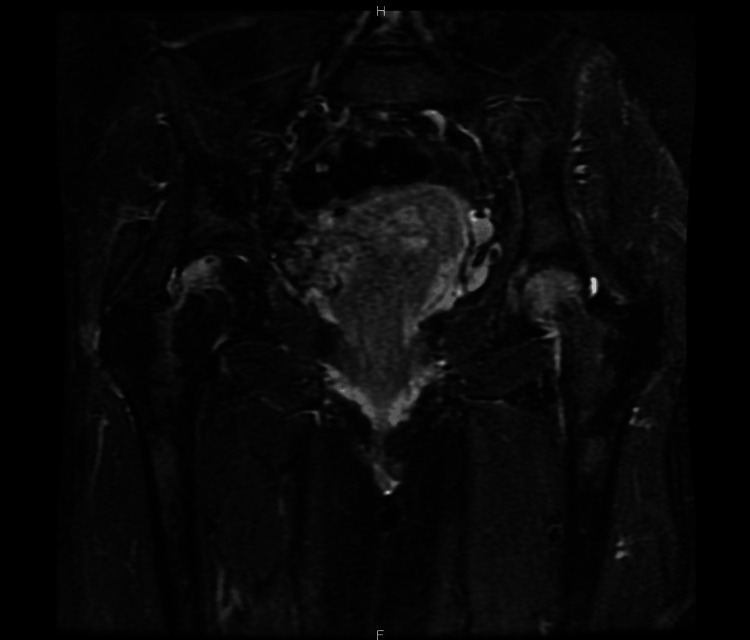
Coronal T2‐weighted MRI of the pelvis Both femoral heads show hyperintense bone marrow edema (arrows), a hallmark of transient osteoporosis of the hip. The patient was at 35 weeks of gestation when the scan was performed.
